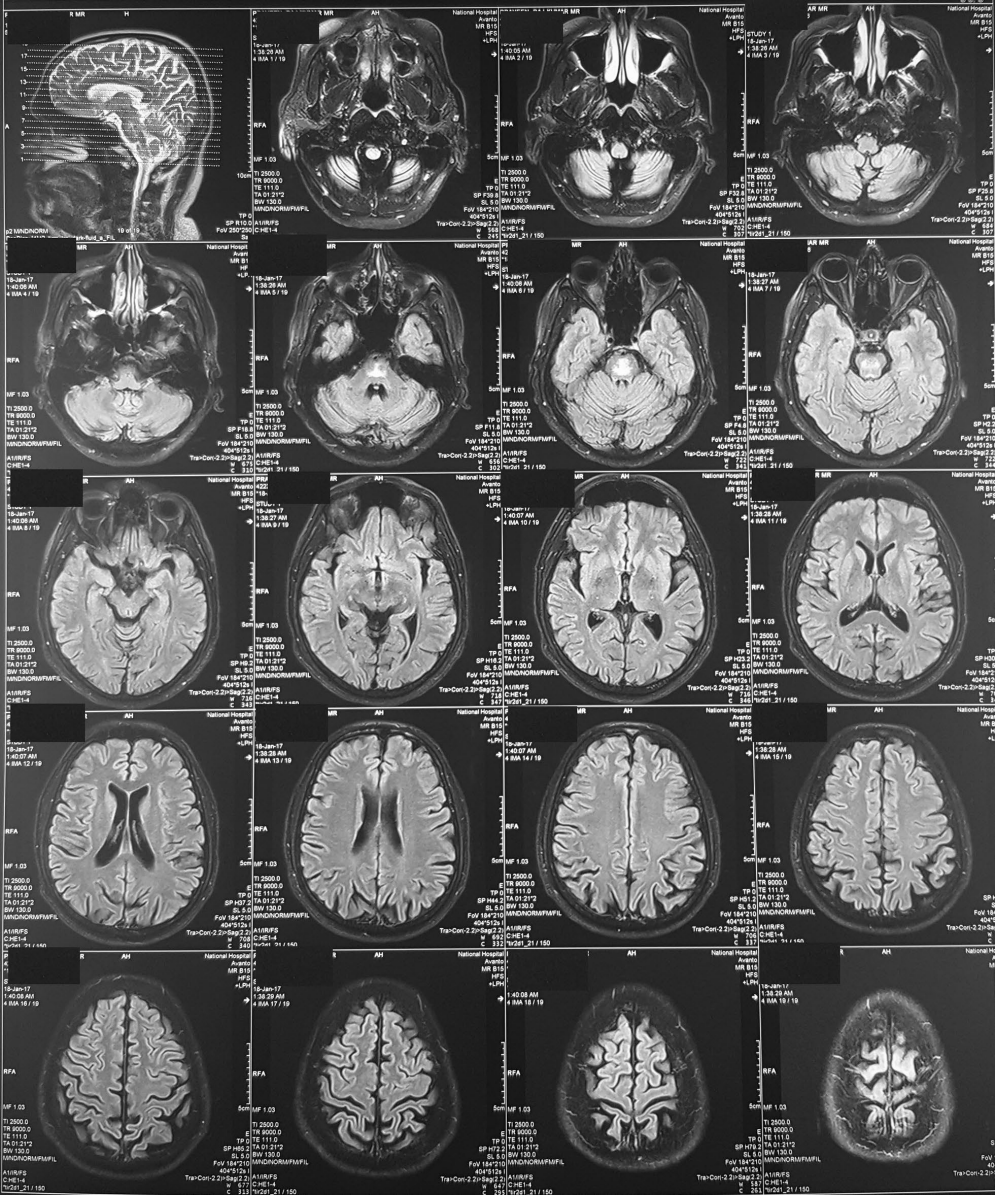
MRI, done 1 month after the first MRI, showing FLAIR high intensity in the central pons with resolution of the changes in the basal ganglia
